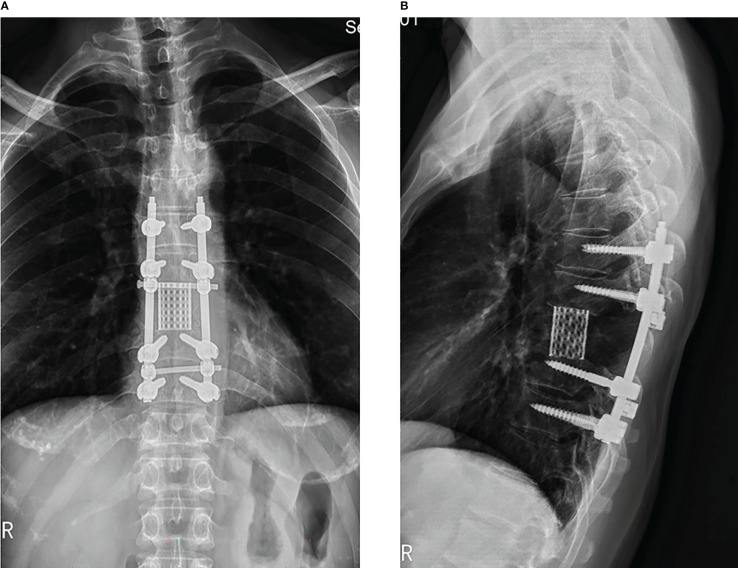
3 months follow-up after the surgery, x-ray display of thoracic spine showing transpedicular screw and a titanium mesh were used in the reconstruction of the stability of the spine in patient. (A: orthotopic position, B: side position).
